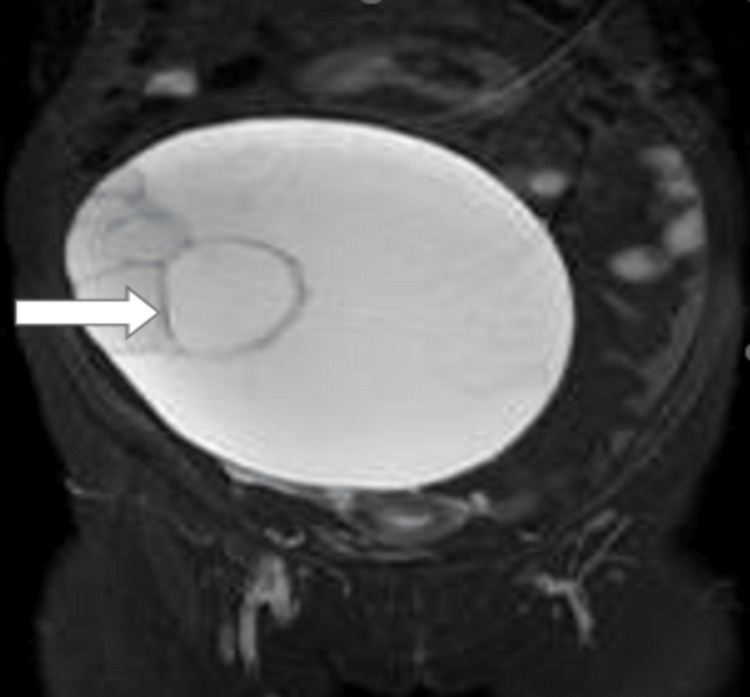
MRI coronal STIR image showing a well-defined hyperintense cystic lesion in adnexa with multiple septations (arrow). STIR: short Tau inversion recovery sequence.
